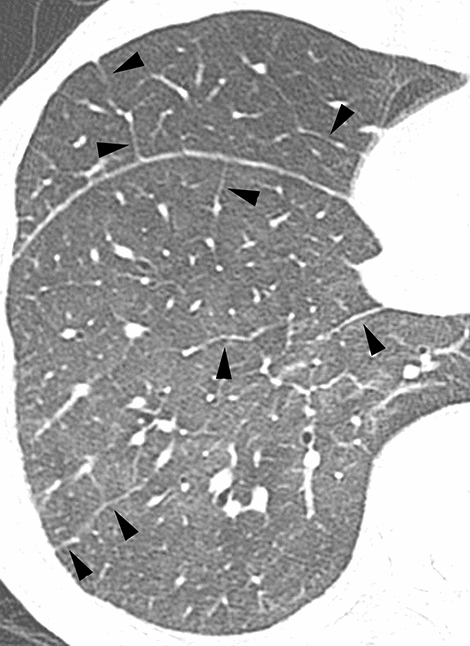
Drug-induced lung injury in a 65-year-old male patient with multiple myeloma.HRCT image of the right lower lung showing extensive GGO and ILS thickening (arrowheads). Note also that nodules are not evident
